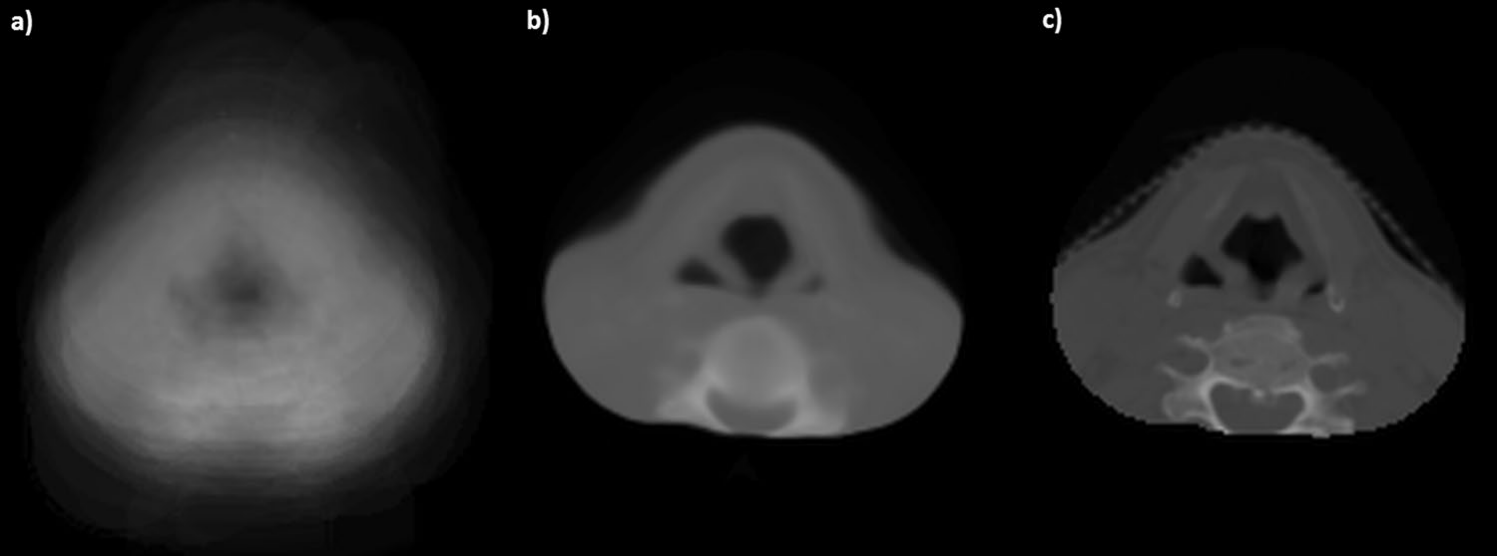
Elastic image registration (EIR) results. Average of the patient population computed tomographic scans (a) before EIR and (b) after EIR, compared to (c) the corresponding reference patient’s scan.
